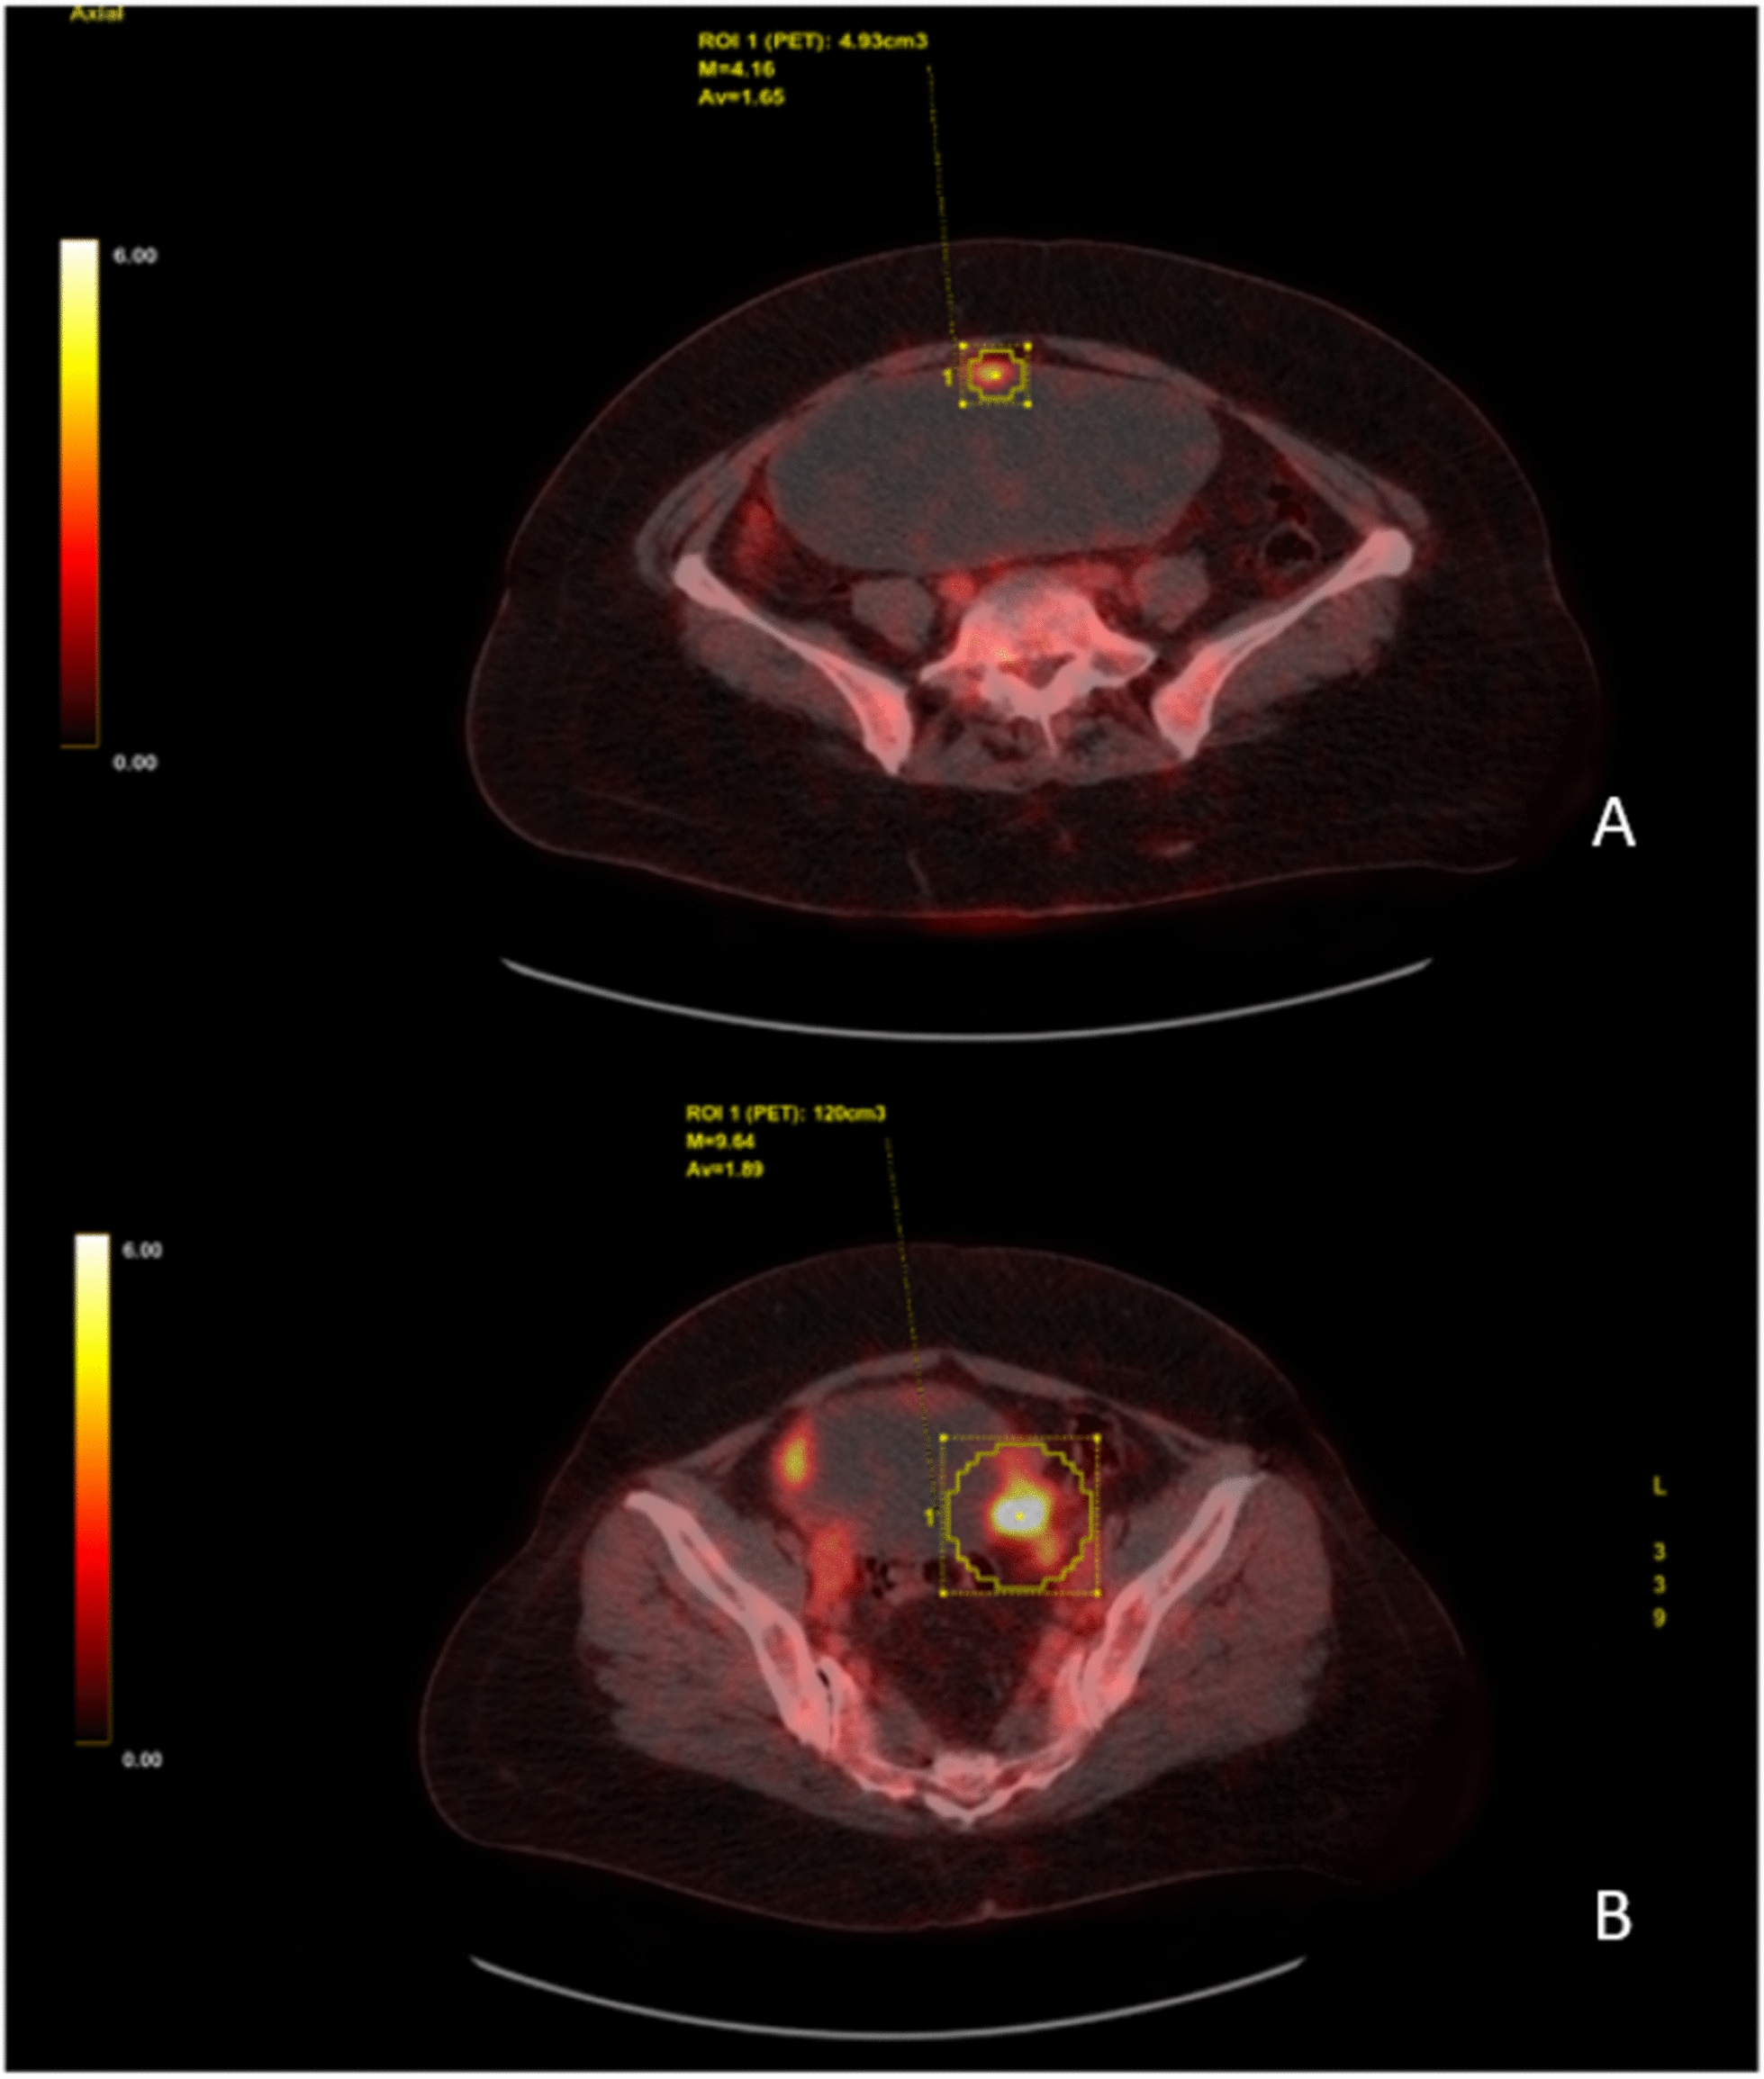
Positron emission tomography–computed tomography scan. A Tumor mass with cystic-liquid parts in lower abdomen presenting with elevated glucose utilization at the margins. Pathological results revealed an endometriod borderline tumor with transition into a moderate degree endometriod ovarian cancer. B Hypermetabolic mass para-iliacal on the left side. Pathological results revealed RDD
